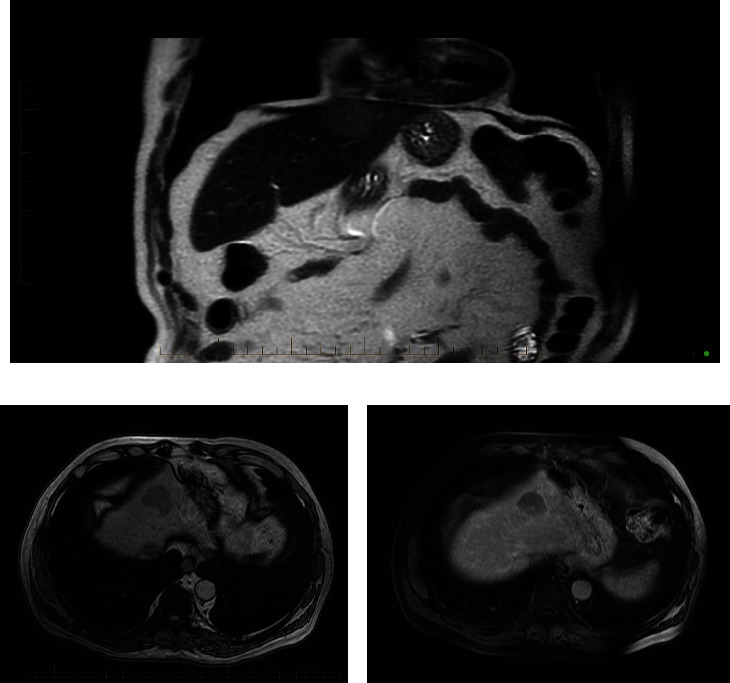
(a–c) MRI of the abdomen. Coronal T2 HASTE image (a) demonstrates a round focus of subtly increased T2 signal in the anterior left hepatic lobe (arrow). Axial gradient echo opposite phase image (b) demonstrates a round focus of decreased signal in the anterior left hepatic lobe (arrow). Axial fat-suppressed T1 image (c) shows the lesion with portal venous washout represented by decreased enhancement in comparison to the surrounding healthy hepatic parenchyma, a feature suspicious for malignancy. Of note, this hepatocellular carcinoma did not demonstrate the more typical arterial enhancement.
